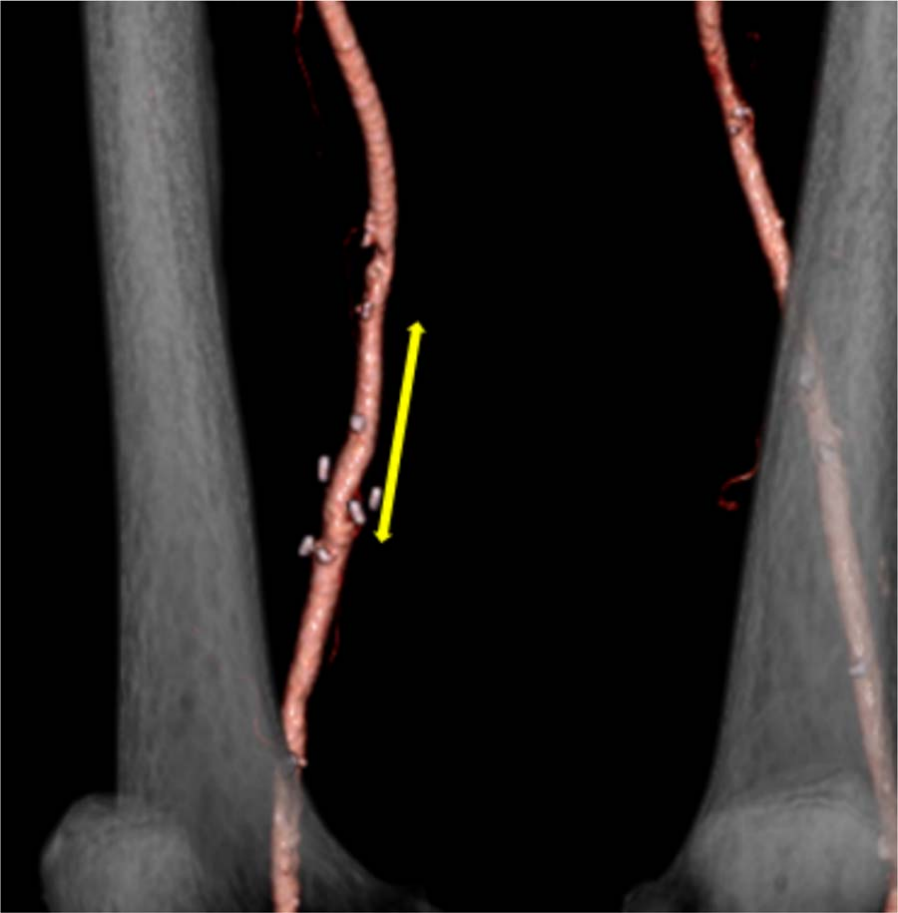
Postoperative contrast-enhanced CT revealed no aneurysm or stenosis formation at the reconstructed site (arrow) and optimal visualization of distal vessels.
